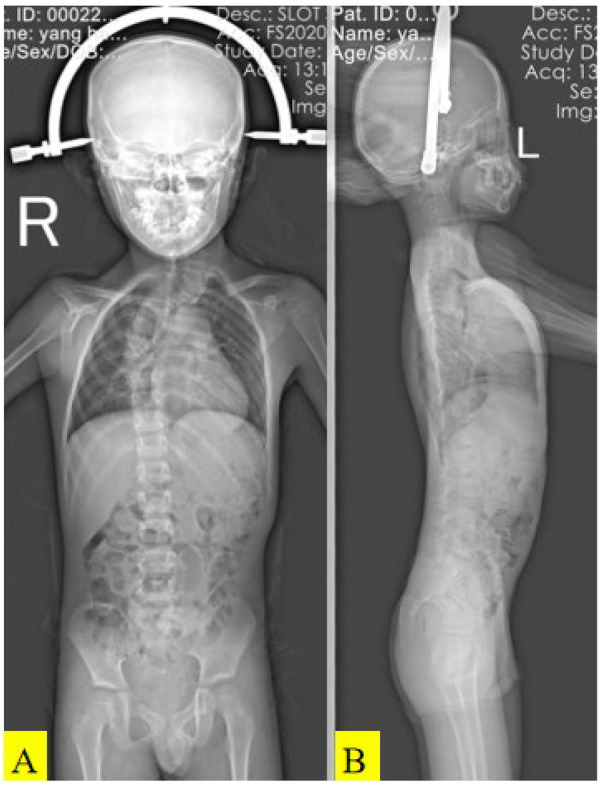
Anteroposterior and lateral X-ray images of the patient's spine in the traction position (A, B).
